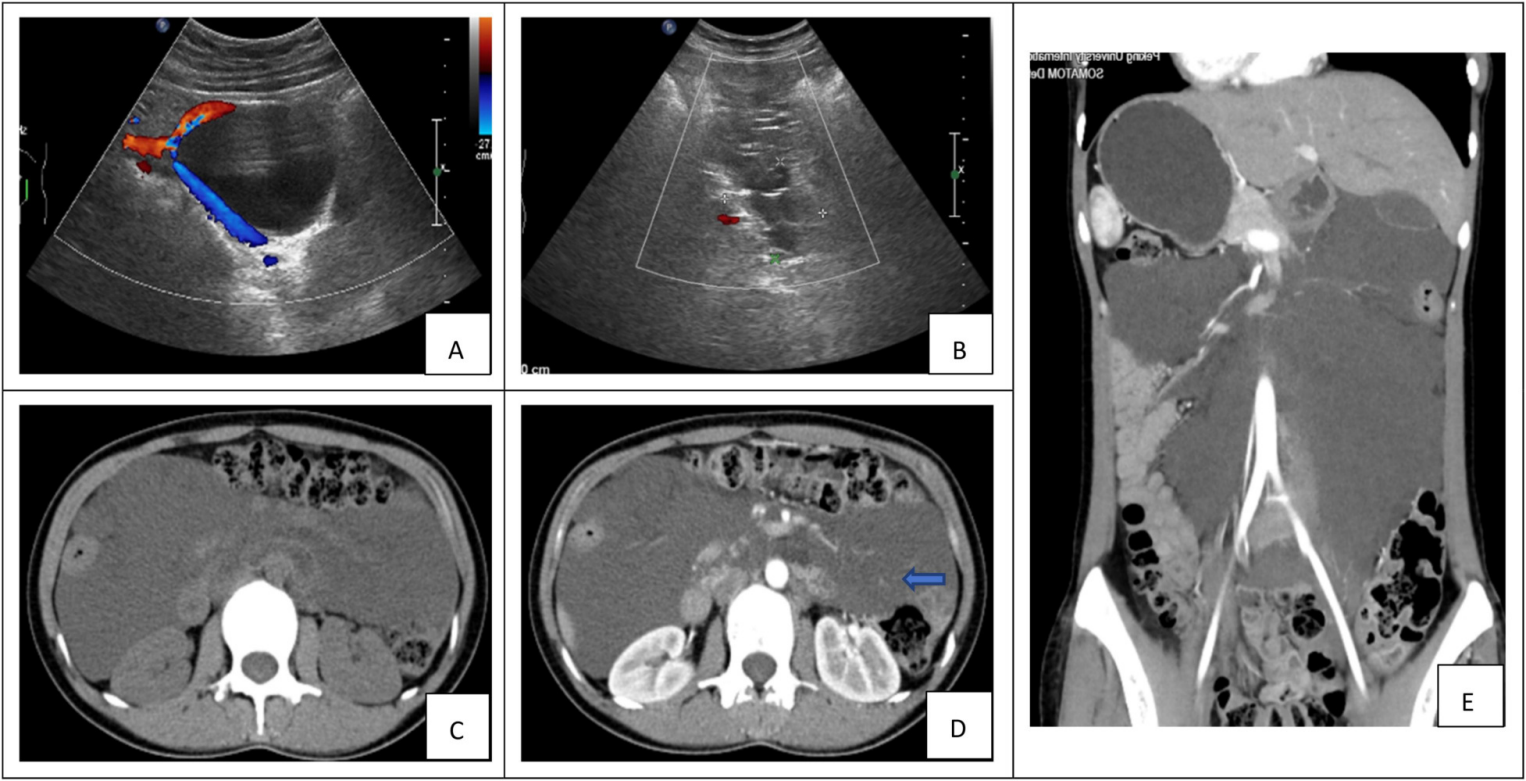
(A–E) Preoperative imaging of retroperitoneal lymphangioma. (A,B) Ultrasonography. (A) A well-circumscribed, oval, anechoic cystic mass is observed at the angle between the left retroperitoneum and the iliac vessels, demonstrating no internal vascular flow on Doppler imaging. (B) Image of a right retroperitoneal lesion reveals multiple thin-walled cystic spaces without calcification. (C–E) Computed Tomography (CT). (C) Axial plain CT scan demonstrates an irregular fluid-attenuation mass with internal septations and partially defined borders. (D) Contrast-enhanced axial CT shows mild enhancement of the septa (blue arrow). (E) Coronal reformatted CT reveals a large cystic lesion causing mass effect on adjacent organs.
